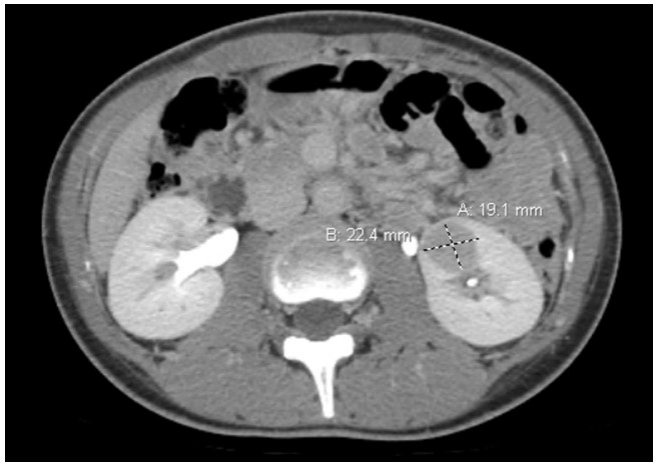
Abdominal CT scan, axial plane, demonstrating the left renal mass.
